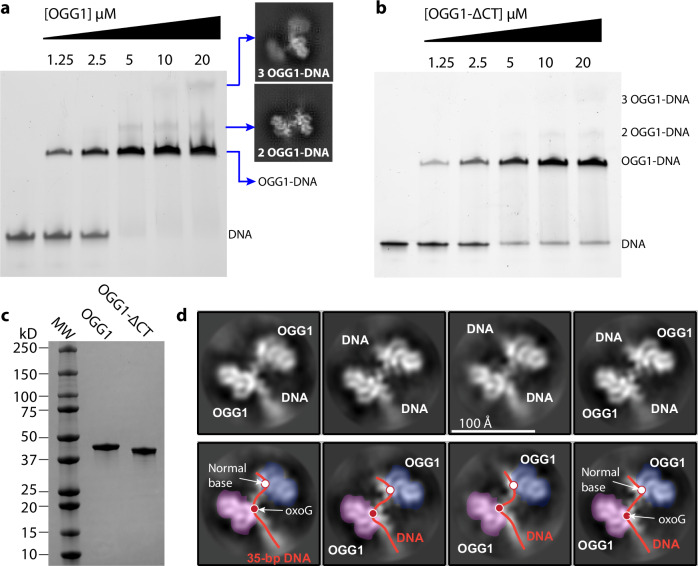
More than one OGG1 can bind to the single 8-oxoG-containing DNA, and the binding ability is reduced in the C-terminus truncated OGG1. a Formation of the OGG1-DNA complex was monitored by EMSA. 2.5 μM of 35-mer DNA duplex containing 8-oxoG at the middle base was mixed with OGG1 at the indicated concentrations. b EMSA was carried out with 8-oxoG DNA duplex using C-terminus deleted OGG1 (OGG1-$$\triangle$$CT) at the indicated concentrations. c SDS-PAGE gel of purified OGG1 and OGG1-$$\triangle$$CT used in the EMSA assay. d Four selected 2D class averages of two OGG1 molecules bound to the same linear DNA. The 35-bp dsDNA is bent twice, at the middle 8-oxoG (red dot) and near the end with a normal DNA base (white dot).
